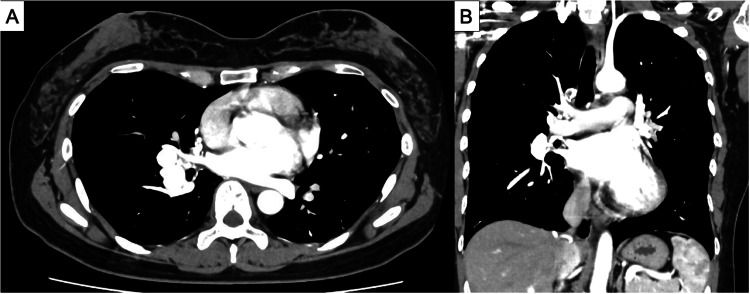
Preoperative contrast enhanced computed tomography (CECT) (A,B) An aneurysm is observed on the ventral side of the right lower pulmonary artery, and the aneurysm communicates with the right lower pulmonary artery.
